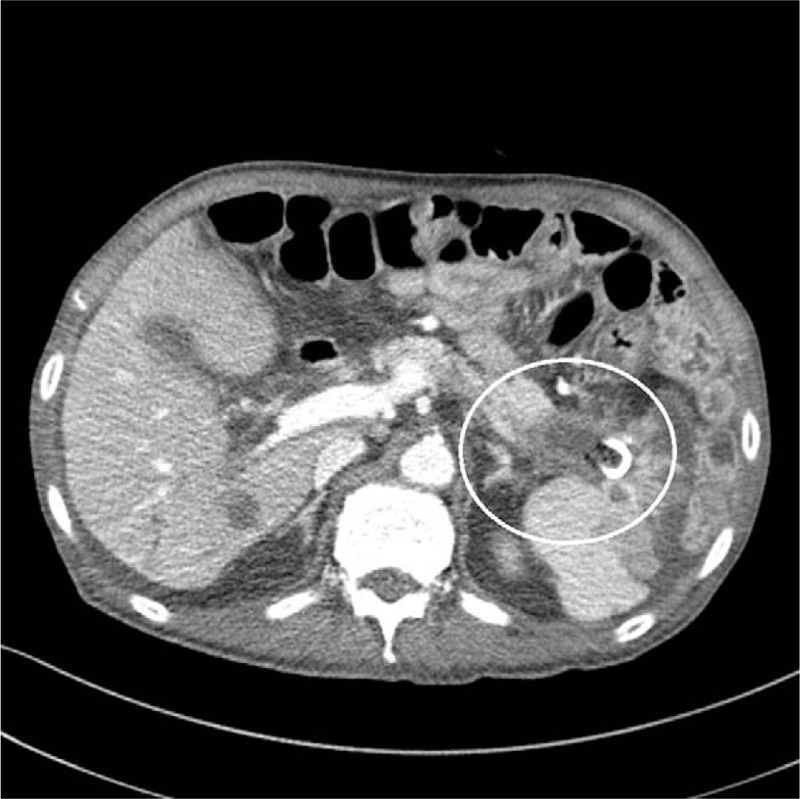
Abdominal CT shows the decrease from 63 to 41 mm in the mass (white circle) in the pancreatic tail. CT = computed tomography.
